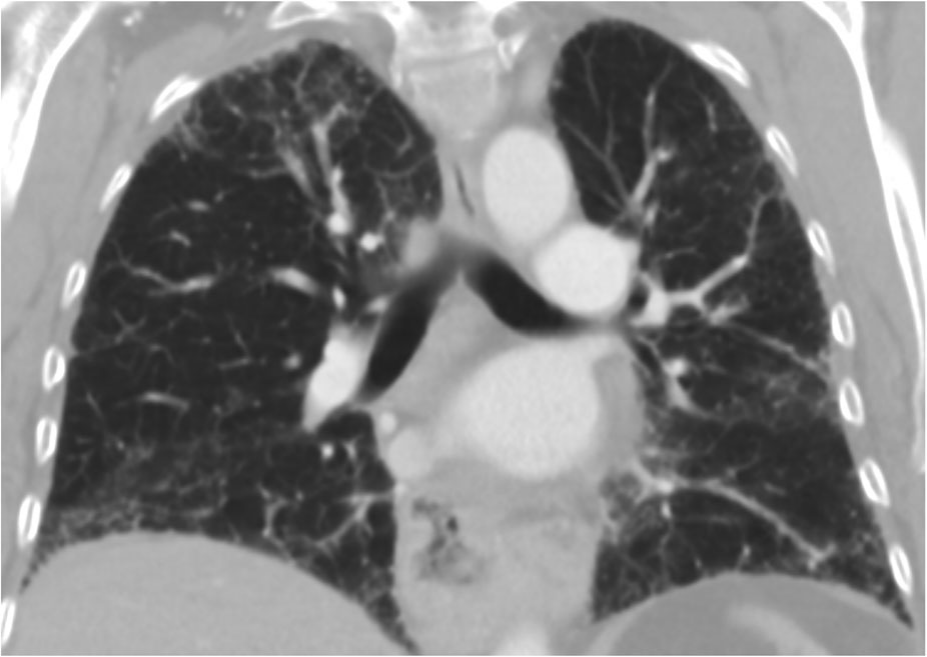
Computed tomography (CT) scan (coronal section) showing fibrotic changes in the posterior and basilar part without any honeycombing.
